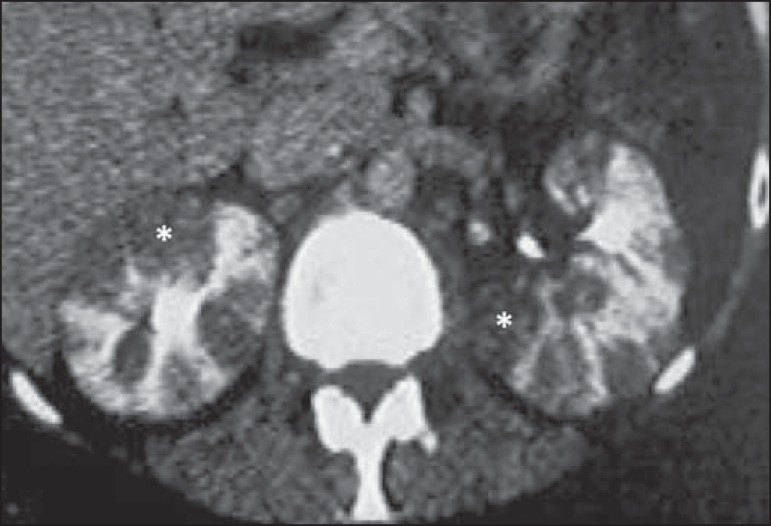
Primary renal lymphoma. Contrast-enhanced CT of the abdomen showing
